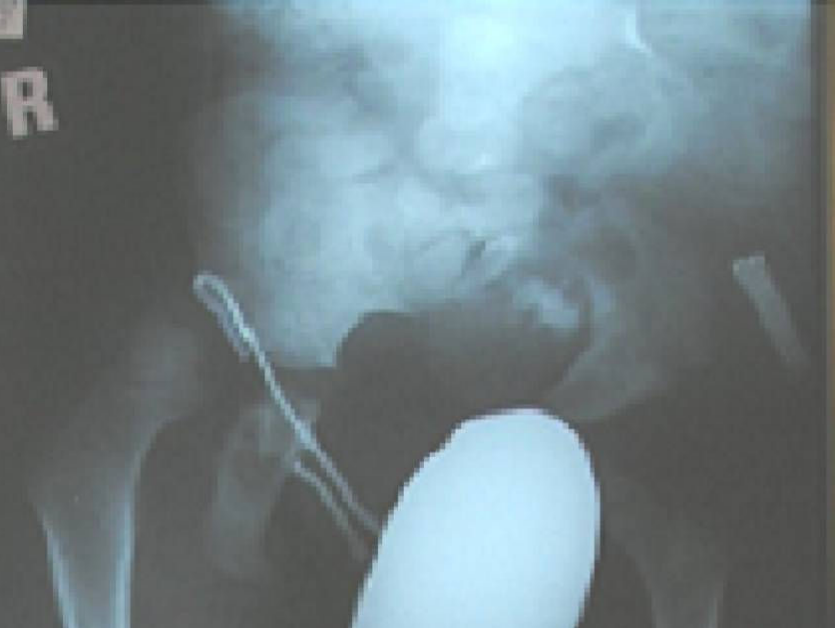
Anteroposterior pelvis radiograph showed total agenesis of the sacrum with subtotal lumbar agenesis. The lowest lumbar vertebrae were resting above an iliac amphiarthrosis.
